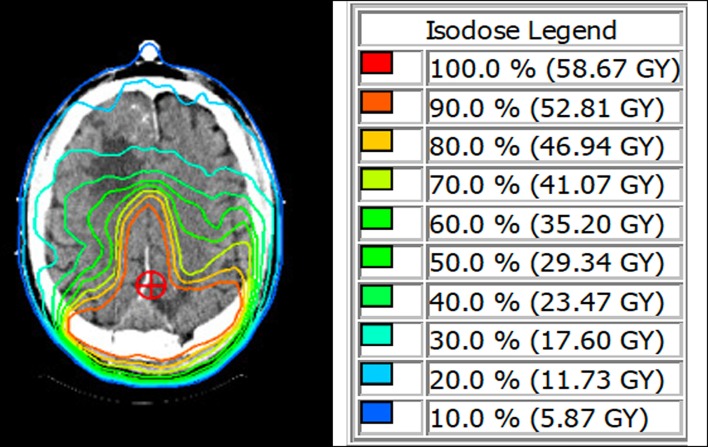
(Left) IMRT treatment planning image. Isodose lines are displayed on post-operative axial CT scan. The targeted total dose (red) is shaped around the remainder of the posterior parasagittal meningioma. The right frontal meningioma can be seen within the lighter blue Isodose line, indicating a potential overall dose between 11.7 and 17.6 Gy. (Right) Isodose legend with corresponding percent total planned radiation dose and actual radiation dose to be given over 30 fractions.
